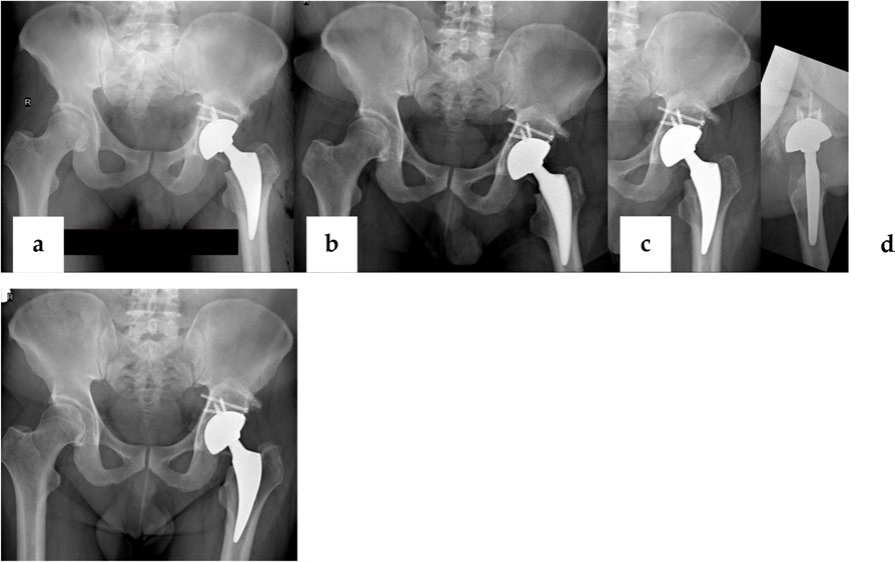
Post-operative radiographs: a immediate, b two weeks, c six weeks, d nine months
